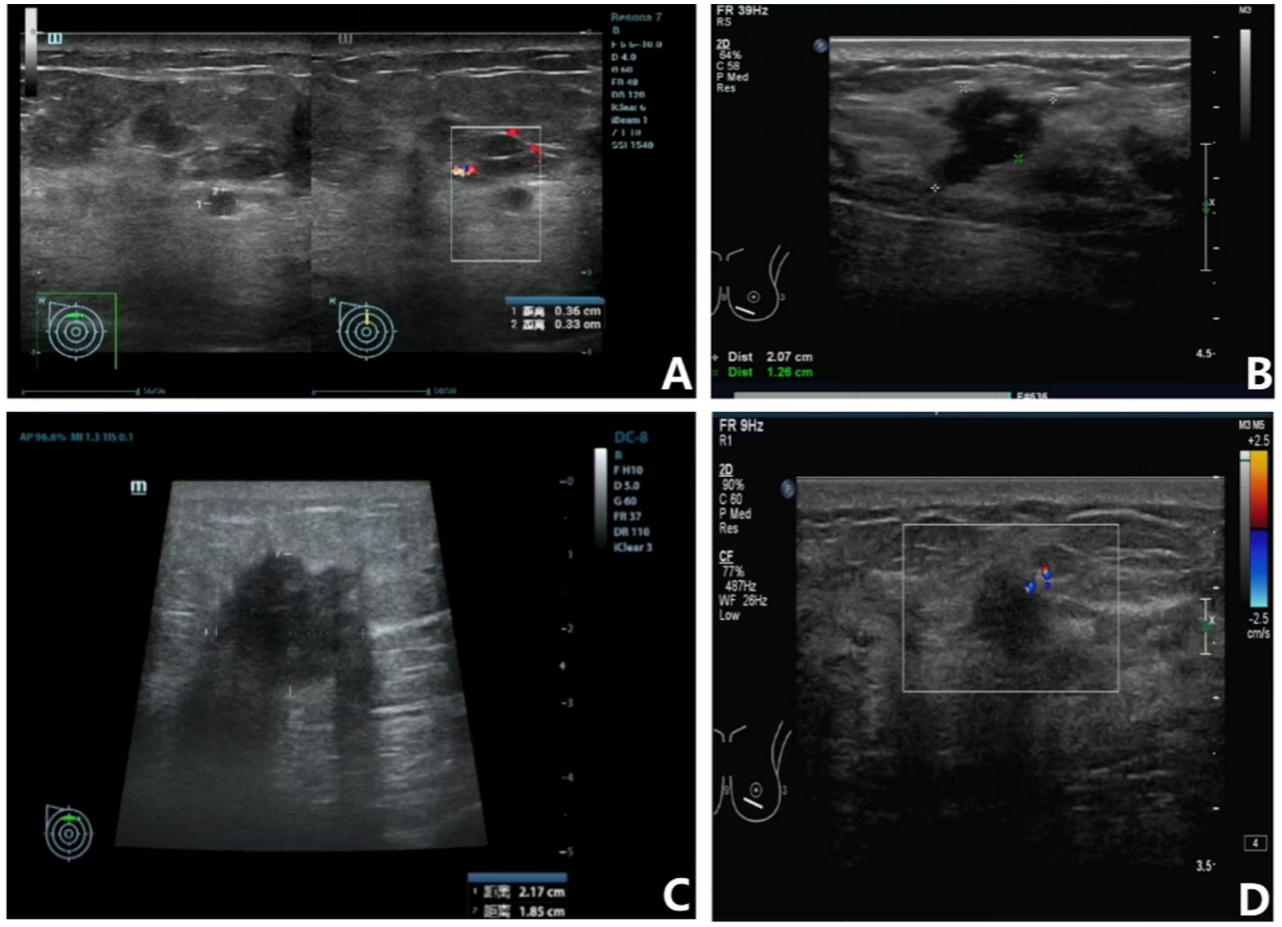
Ultrasound imaging of the breast tumors in the right and left breasts. (A) Pre-NACT ultrasound of the right breast showing a necrotic mass measuring 2.3 × 1.5 cm; (B) Post-NACT ultrasound of the right breast showing a slight increase in tumor size to 2.2 × 1.9 cm; (C) Pre-NACT ultrasound of the left breast revealing a mass measuring 2.1 × 1.3 cm; (D) Post-NACT ultrasound of the left breast showing a reduction in tumor size to 1.2 × 0.9 cm.
